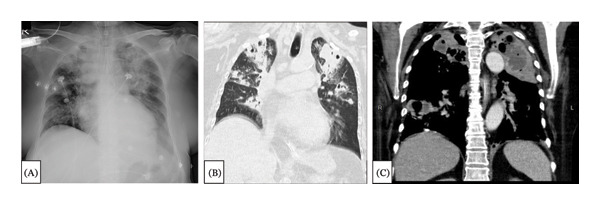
The chest X‐ray on Day 1 showed multifocal ground glass opacities (GGOs) and consolidation over bilateral lungs (A), while the computer tomography (CT) without contrast additionally showed few possible cavitary foci (B). The following CT with contrast on Day 15 showed mild resolution of GGOs yet progressing cavitary foci and air fluid levels (C), suggesting a lung abscess and necrotizing pneumonia formation.
